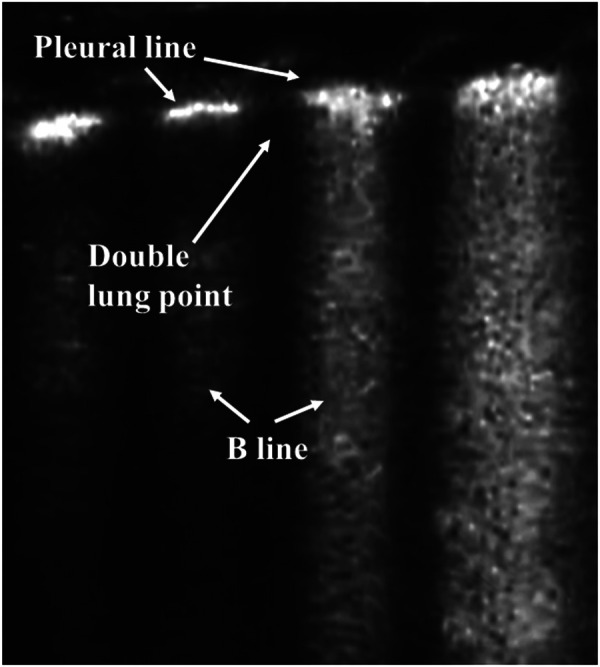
Transient tachypnea of the newborn on lung ultrasonography. Double lung point: there is a sharp change in echogenicity as a result of the abundance of B-lines between the upper and the lower lung fields.
